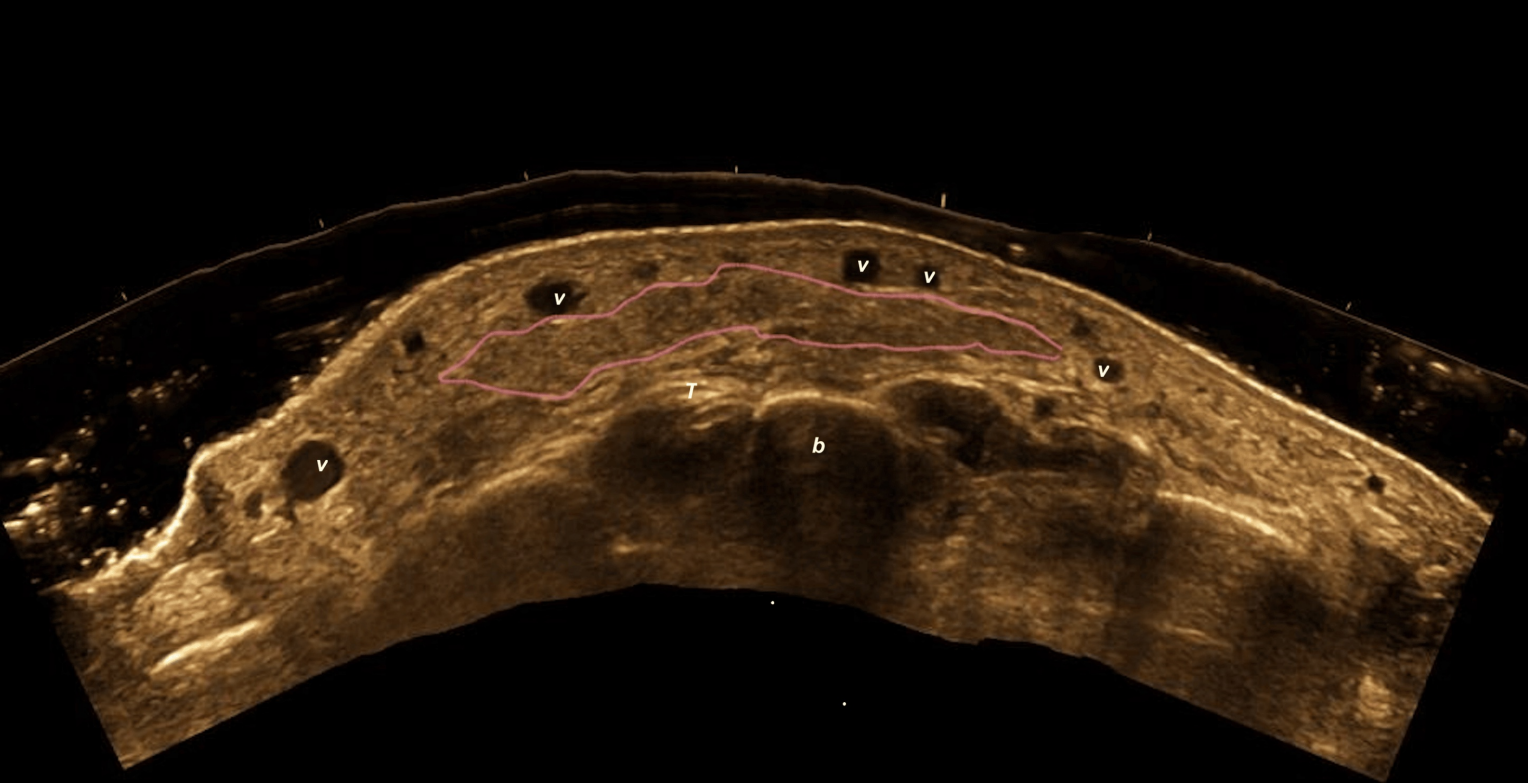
Sonographic appearance of autologous fat grafting in the dorsal hand (transverse view) Pink boundary lines outline elongated, isoechoic adipose deposits consistent with transplanted autologous fat. The superficial venous plexus (v), extensor tendon (T), and bone (b) are labeled.
